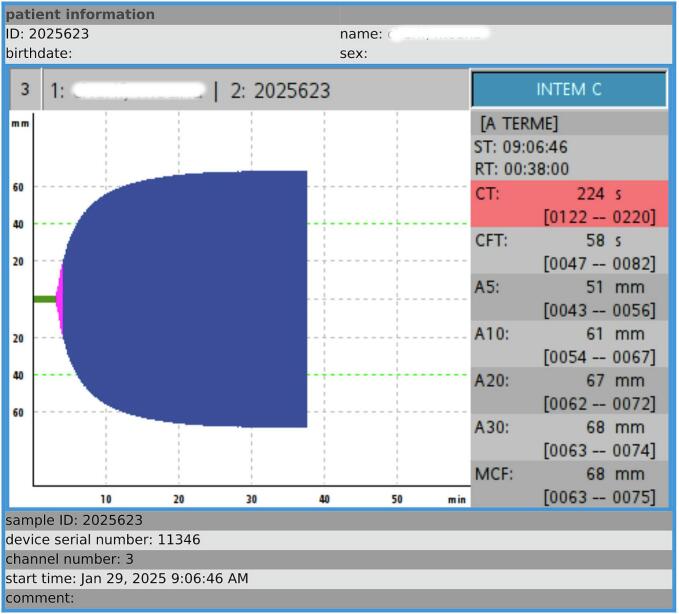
Rotational Thromboelastometry (ROTEM) Traces of the Clinical Case – Postoperative ROTEM (INTEM Module). INTEM: ellagic acid activated intrinsic pathway (CT, clotting time[s]; CFT, clot formation time[s]; MCF, maximum clot firmness [mm]; A5, amplitude time point of 5 min after CT [mm]; A10, amplitude time point of 10 min after CT [mm];
